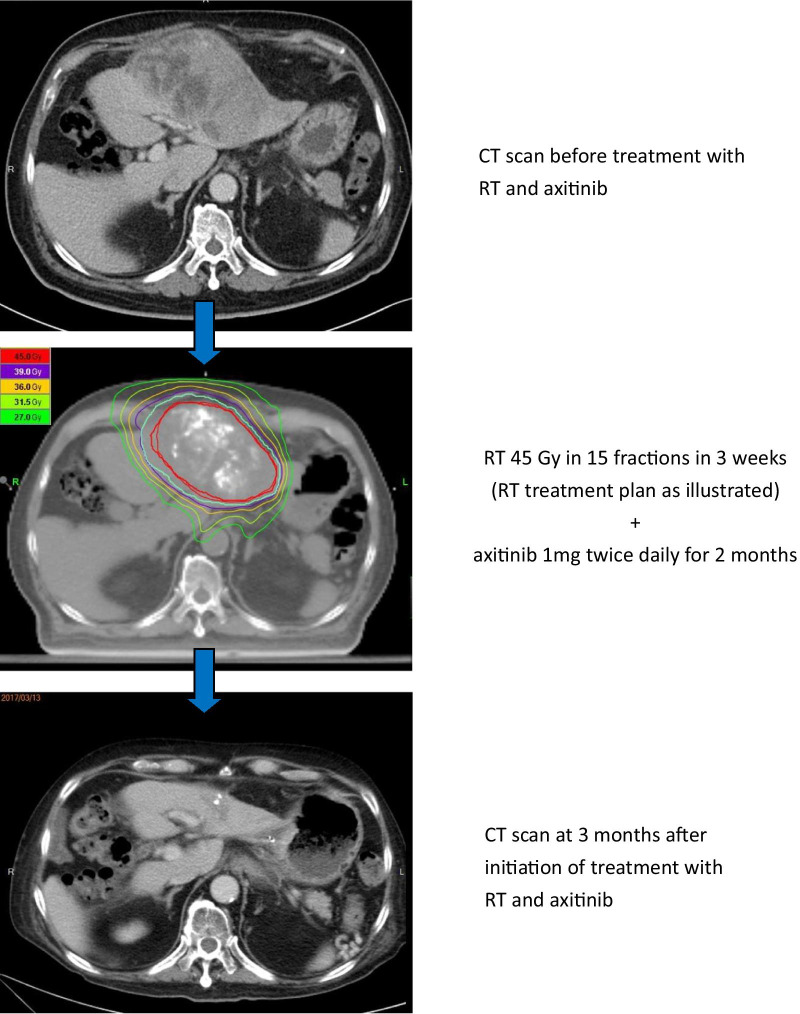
A case presentation (complete response, long-term alive, no recurrence). A 72-year-old man, with liver cirrhosis Child–Pugh class A (non-HBV, non-HCV related), was diagnosed as having a 12-cm HCC in left lobe of liver with classical enhancement pattern and involvement of left portal vein by CT scan in August 2016, clinically staged as cT3bN0M0, BCLC stage C. High alpha-fetoprotein (AFP) up to 160.1 ng/ml was noted. TACE failed with only partial obliteration of tumor vessels. He was then eligible for this phase I trial. He received RT with 45 Gy in 15 fractions plus axitinib 1 mg twice daily for 2 months. The patient tolerated the treatment well. At 3 months after RT initiation, follow-up CT scan revealed complete response of the tumor, and AFP decreased to 1.9 ng/ml. In 2020, the patient is still regularly followed up without recurrence
